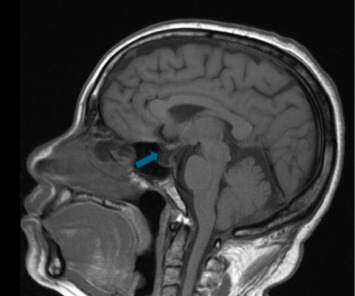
Brain MRI. Sagittal T1 without contrast showing thin enhancing tissue along the floor of the pituitary fossa with cerebrospinal fluid occupying the rest of the fossa suggesting a partially empty sella. Blue arrow points toward empty sella.
